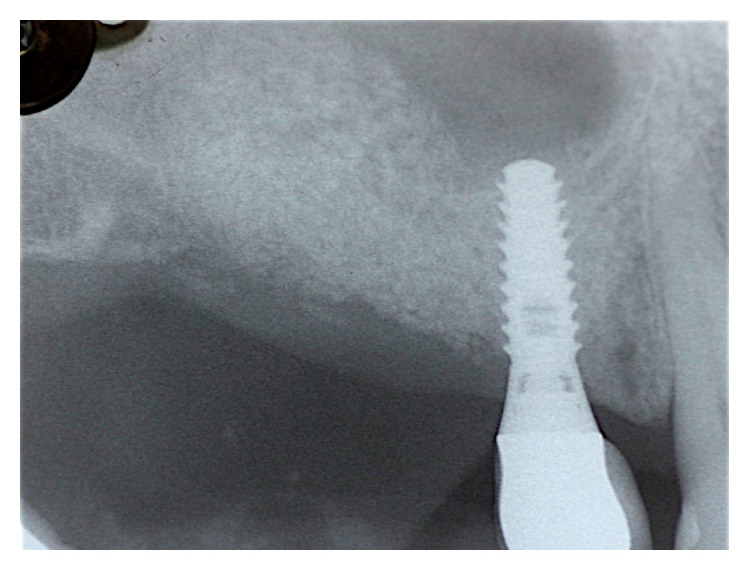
Radiograph 6 months after surgery.
